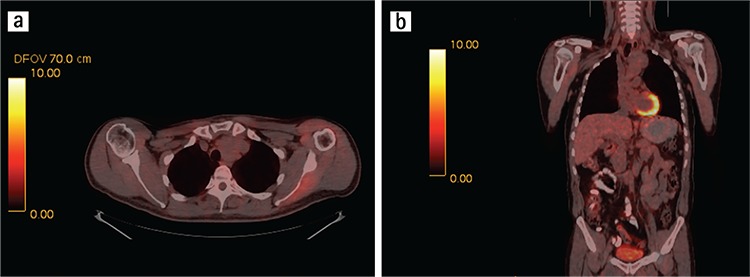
Axial and coronal PET-CT slices of a solitary fibrous tumor located in mediastinum which slightly compressed trachea.
